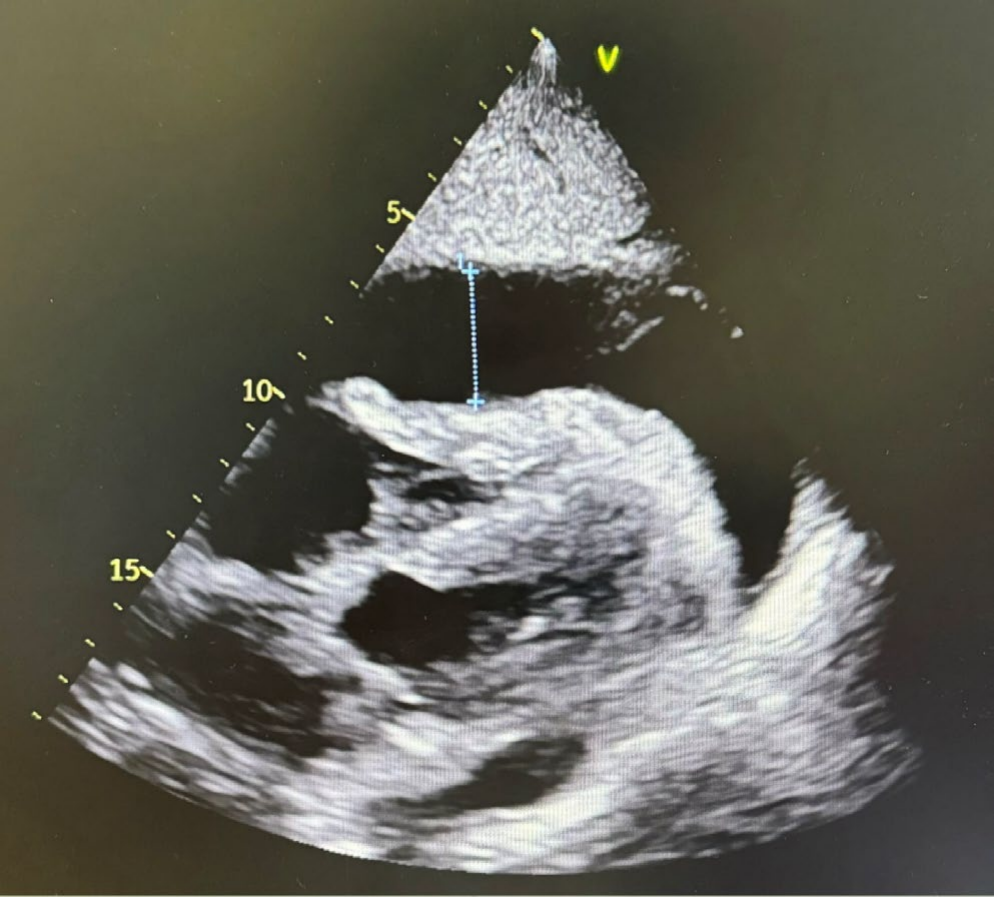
CT scan and injection of contrast into the implantable CVAD appearing in the pericardium. Confirmation of the fistulization of the catheter into the pericardium.
